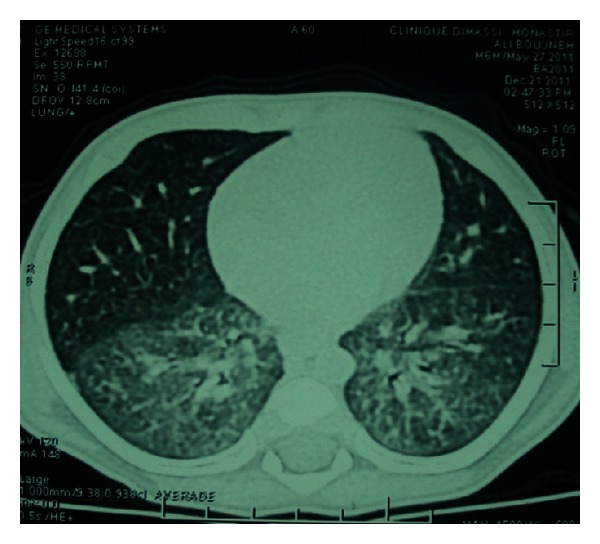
Chest computed tomography: bilateral interstitial infiltrate.
